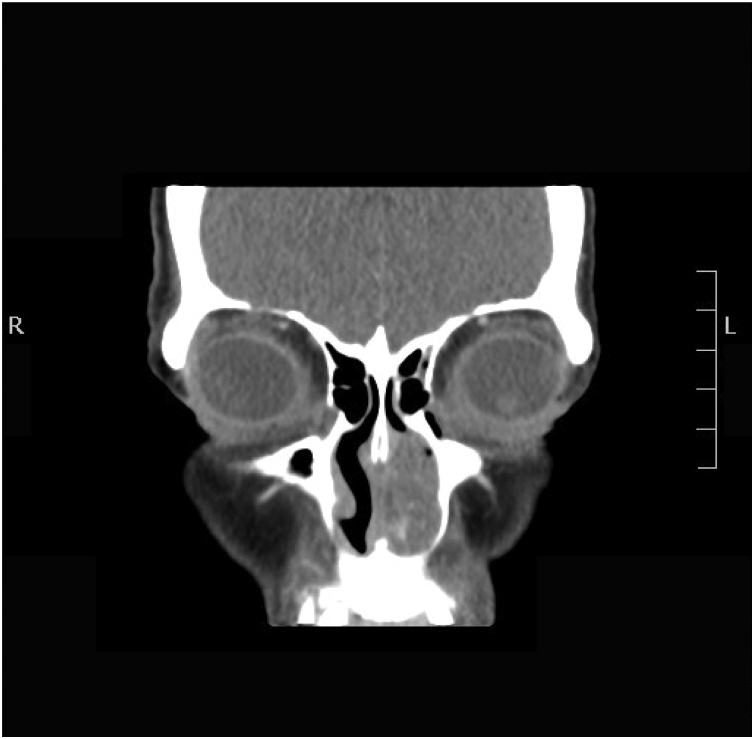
CT scan of the paranasal sinuses with contrast. The coronal section showed a left anterior lobulated nasal mass of undetermined origin at the level of the cartilaginous part of the nasal septum with mild heterogeneous post IV contrast enhancement.
